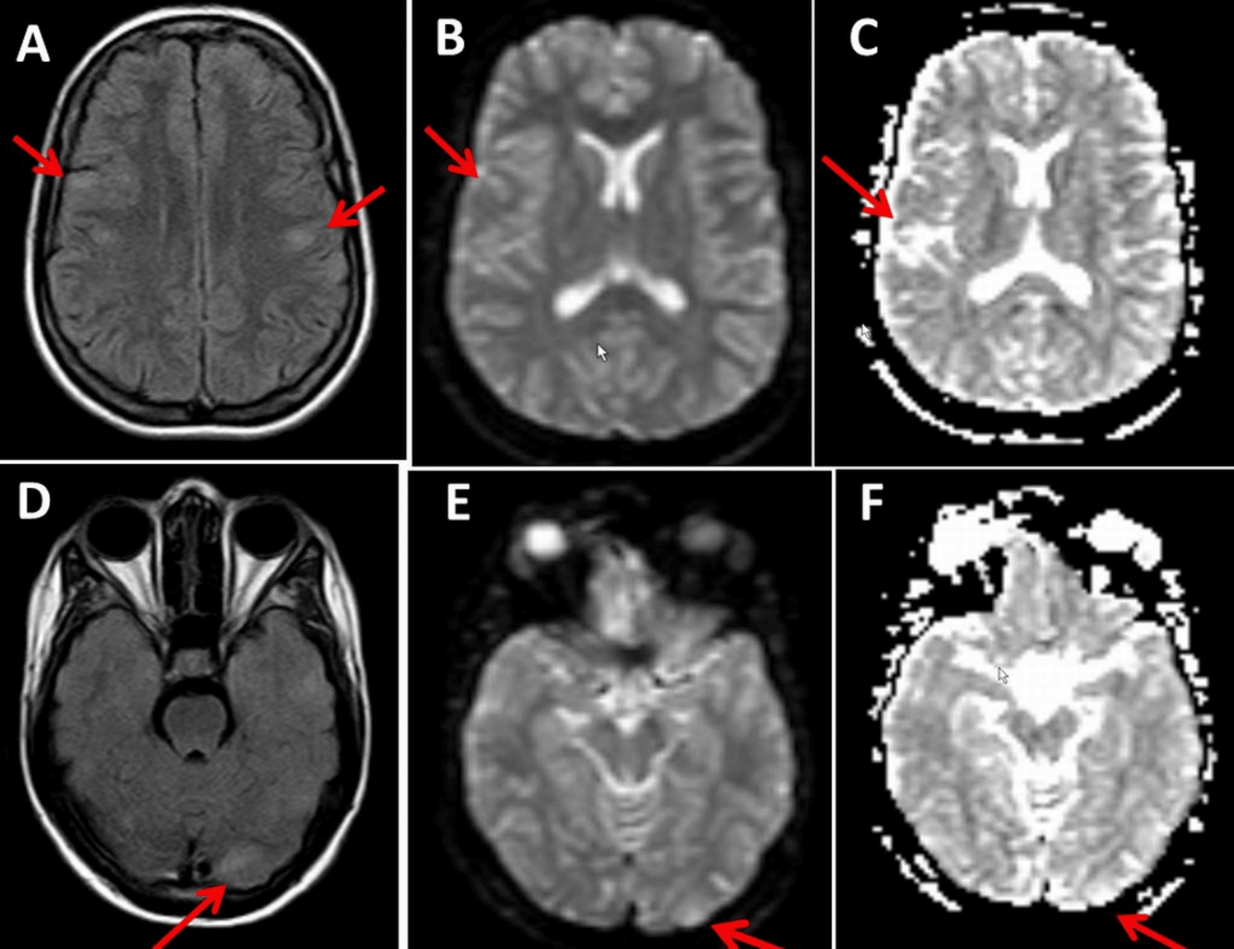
Cerebral MRI images. Cerebral MRI shows vague hyperintense lesions with a diameter of 7 mm in a bilateral parietal distribution subcortically on axial FLAIR (panel A). In addition, a tender hyperintense FLAIR lesion is seen in the left occipital region (panel D), showing mild enhancement in T1 (not shown). These lesions were slightly hyperintense in diffusion-weighted imaging (DWI) (panels B and E) and apparent diffusion coefficient (ADC) images (panels C and F).
